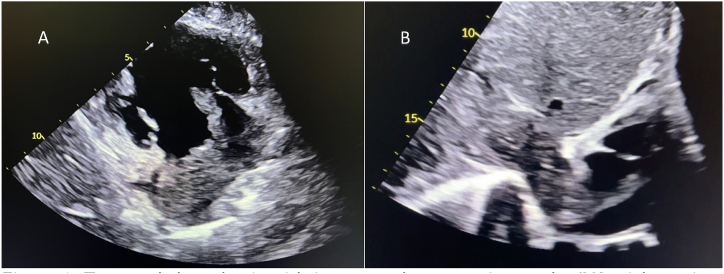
Tumoural thrombosis with intravascular extension to the IVC, right atrium and ventricle on bedside echocardiography. A – parasternal long axis/right ventricular inflow view B- subxiphoid view.
